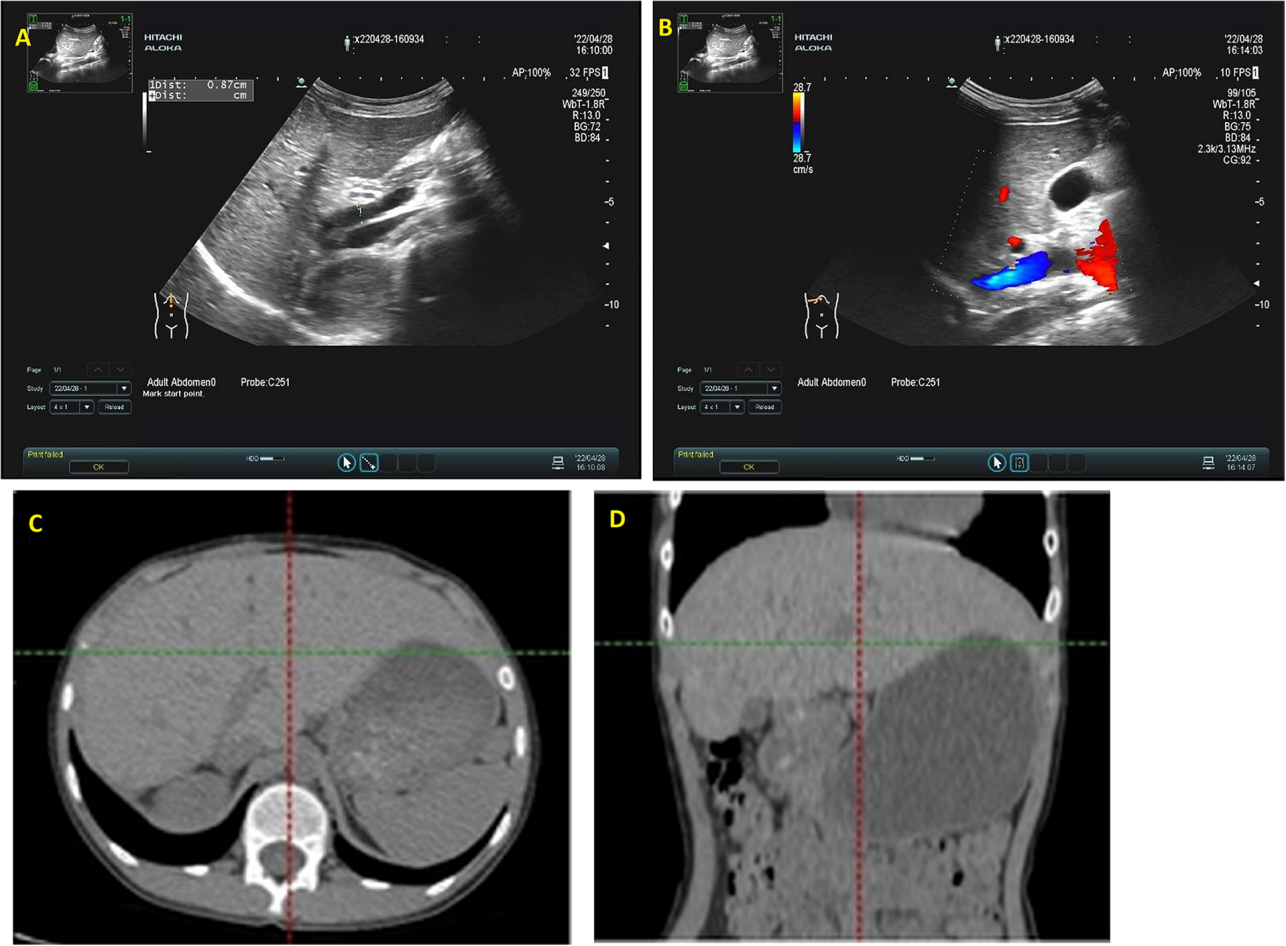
Abdominal ultrasound evaluation and CT examination two months postoperatively. (A) Demonstrates robust blood flow within the intrahepatic portal vein, with no notable abnormal echogenicity; (B) reveals no identifiable communication between the portal vein and the inferior vena cava. (C,D) The liver has regenerated, multiple small nodular shadows can be seen in the lower abdominal cavity.
